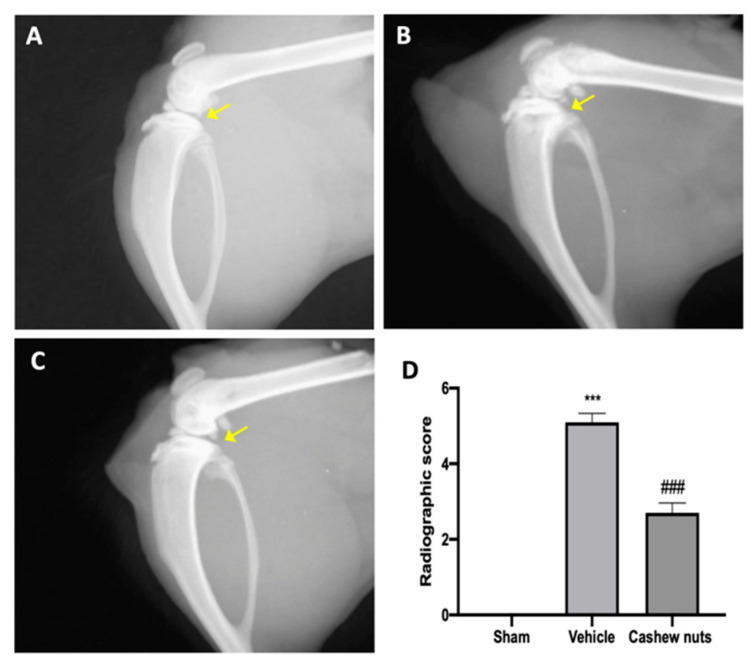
Effect of cashew nuts on radiographic analysis: sham (A), vehicle (B), cashew nuts (C), radiographic score (D). A *p*-value < 0.05 was considered significant. * *p* < 0.001 vs. sham-vehicle, ### *p* < 0.001 vs. MIA-vehicle.
